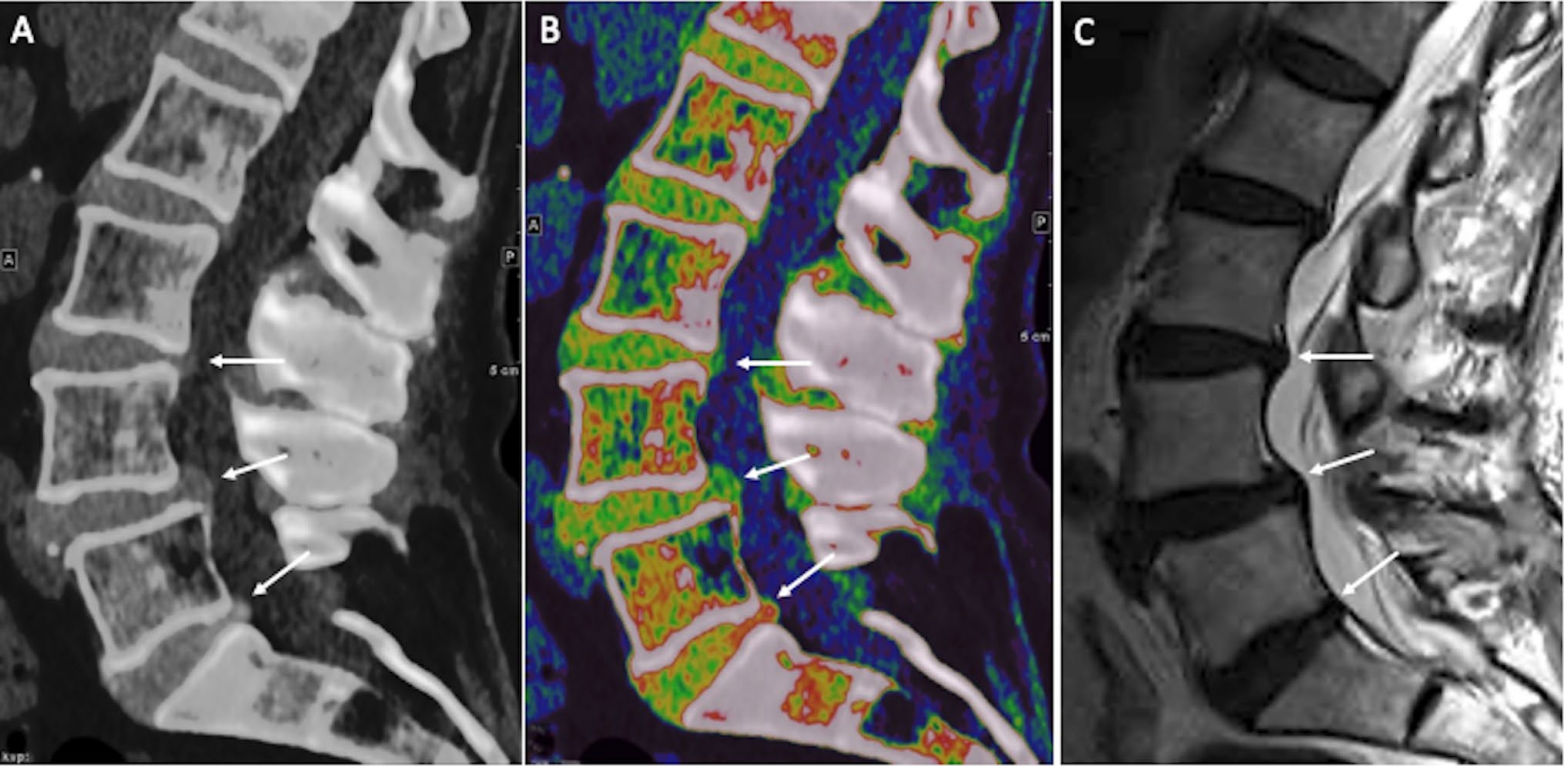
Lumbar spine images of a 58-year-old man with low back pain. Standard sagittal unenhanced CT a show L4/L5 degenerative spondylolisthesis, with L3/L4 and L4/L5 lumbar disk protrusions and L5/S1 disk herniation (arrows). Disk protrusions and herniation are better depicted on sagittal VNCa DECT image (b) and sagittal T2-weighted MRI image (c)
